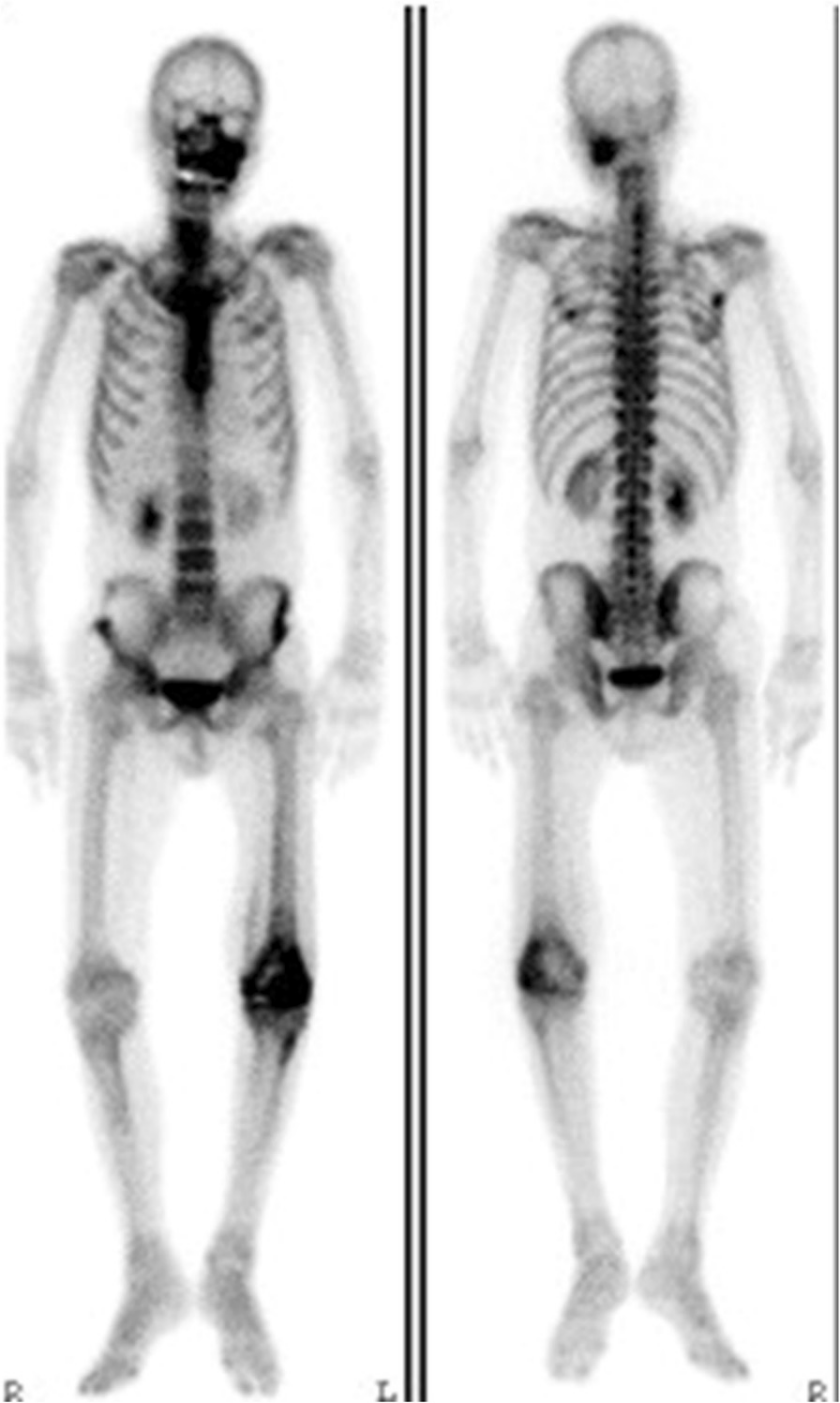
Bone scintigraphy. There is abnormal accumulation in the left distal femur. The abnormal accumulation in the craniofacial bones was attributed to fibrous dysplasia
